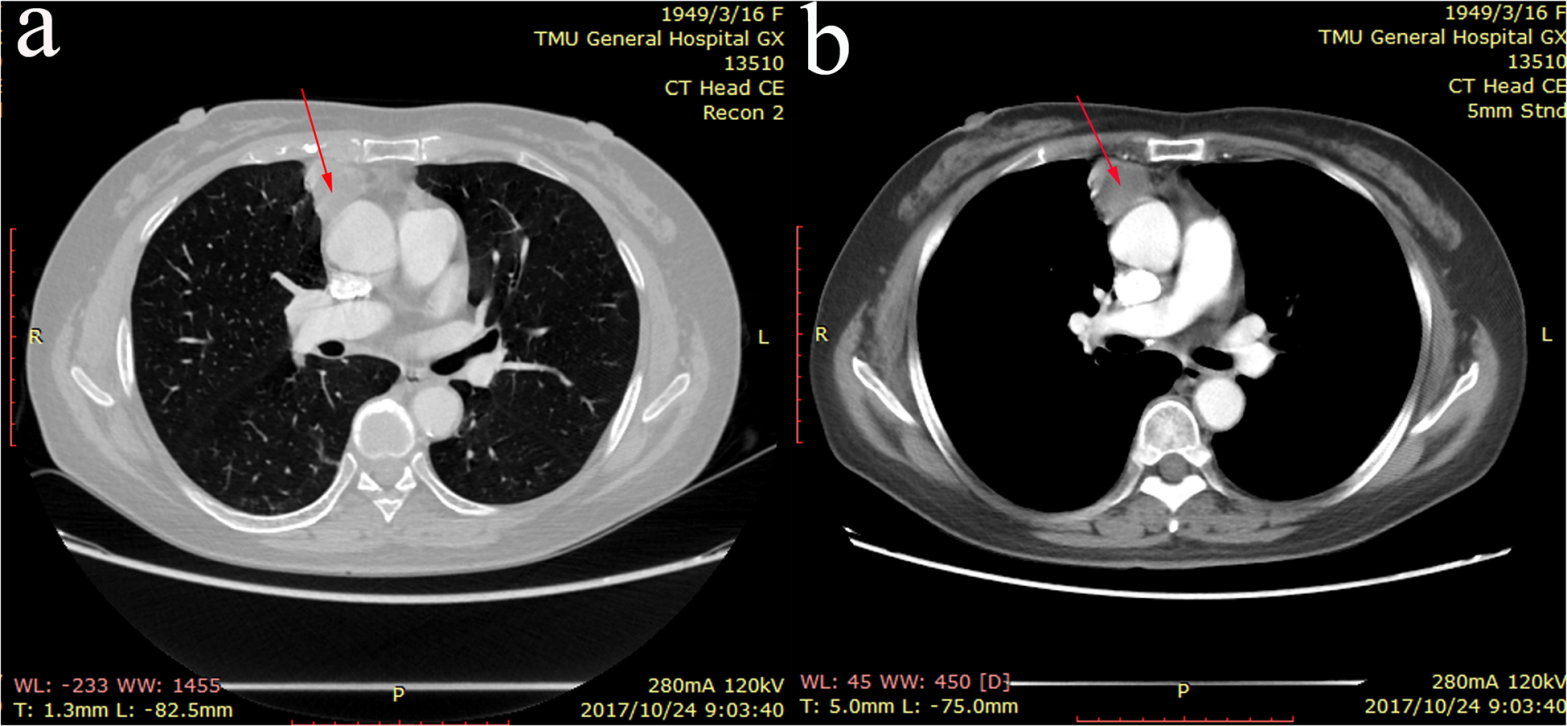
Chest CT scans. Chest CT scans of this patient showed a 27 mm × 28 mm (arrows) mass with mixed cystic solid lump, irregular lobes and fat density features in right upper mediastinum
